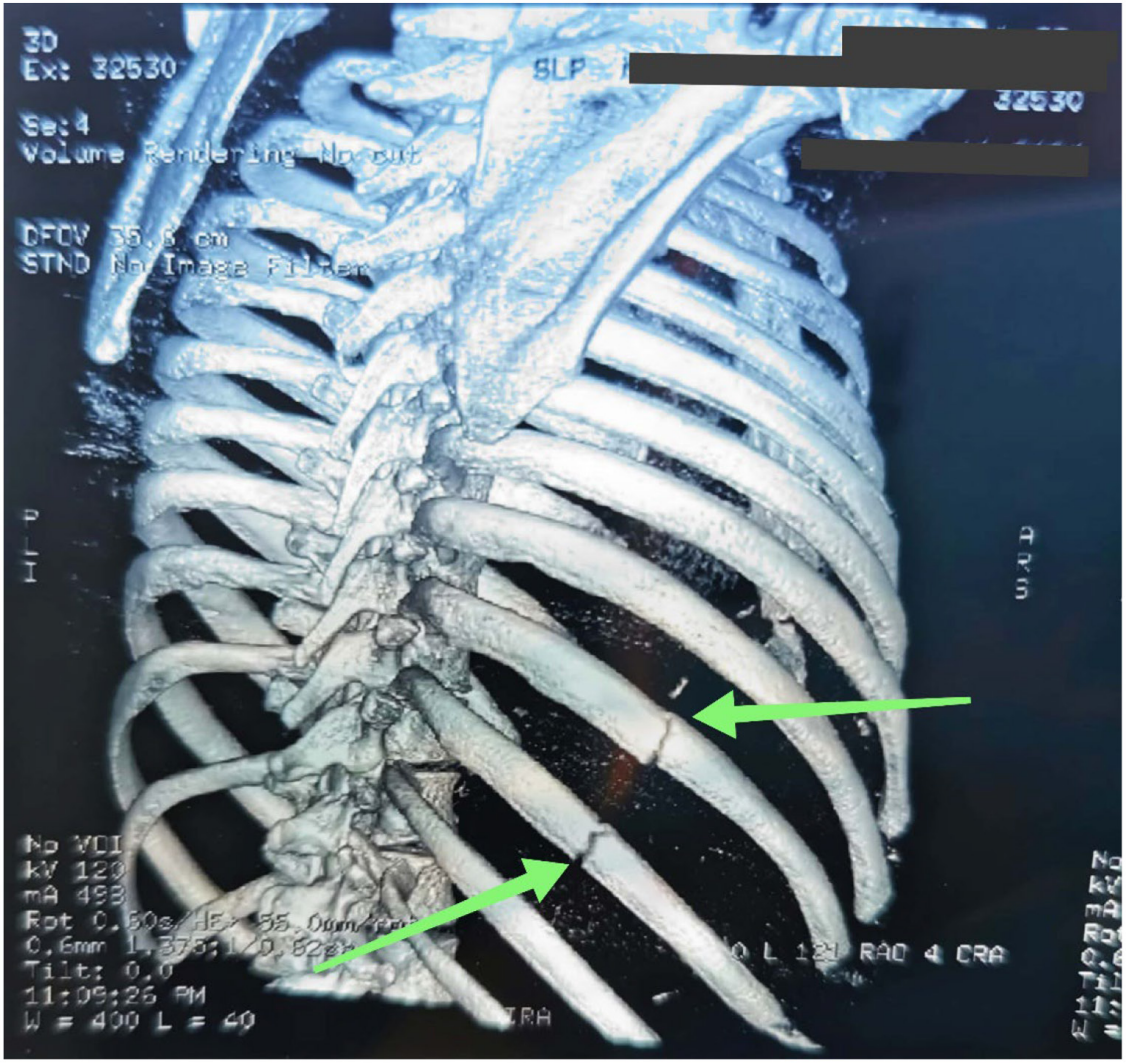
Chest CT 3D reconstruction showing a linear fracture of the right ninth and tenth ribs (green arrows).
